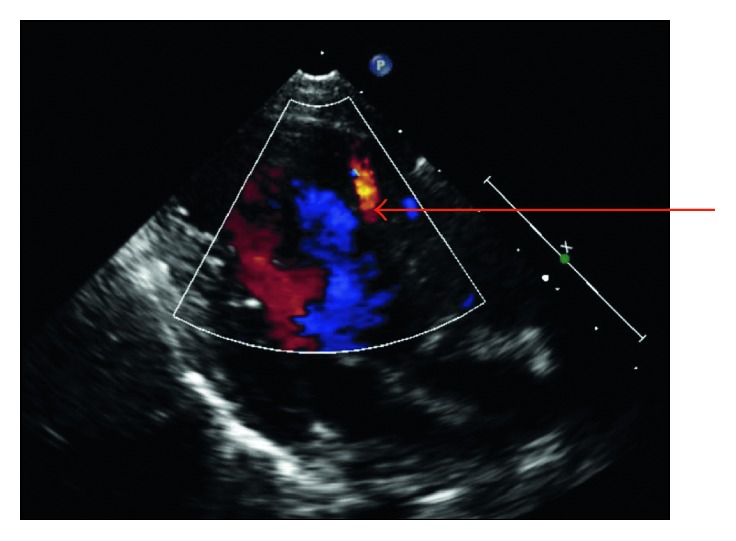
Parasternal long-axis view demonstrating a laminar flow from the LV to the interventricular septum (red arrow).
